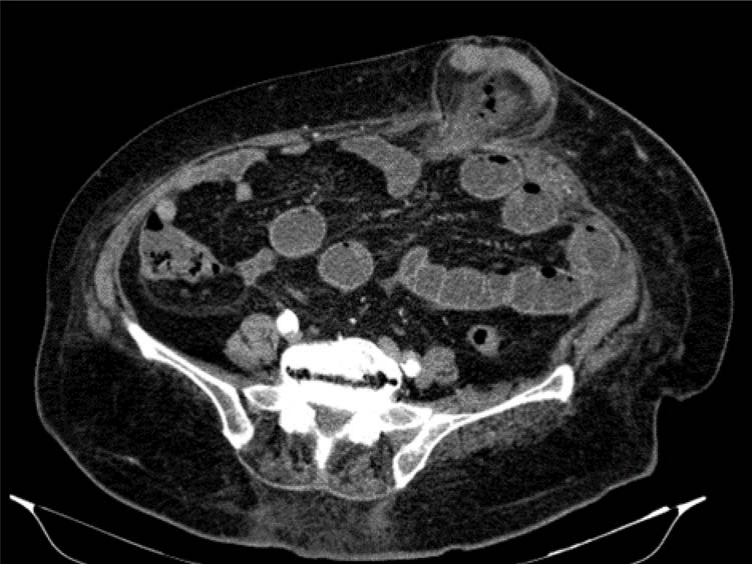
Abdominal CT: hernia content composed of intestinal loop and densification of the mesentery with gas bubbles.
